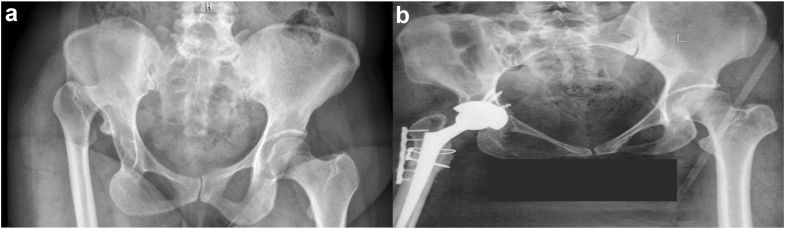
(a) Preoperative and (b) postoperative X-ray of a patient with Crowe IV developmental dysplasia of the hip (DDH). In this patient, due to neurological issues during the femoral trial reduction, it required additional bone resection from the femur before prostheses insertion.
